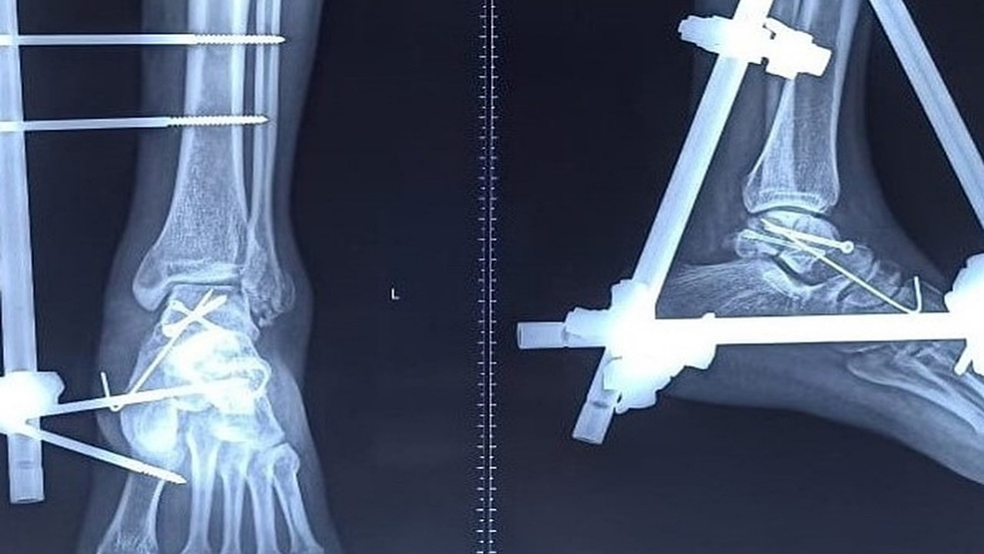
Postoperative X-ray showing fixation of talus with external fixator
